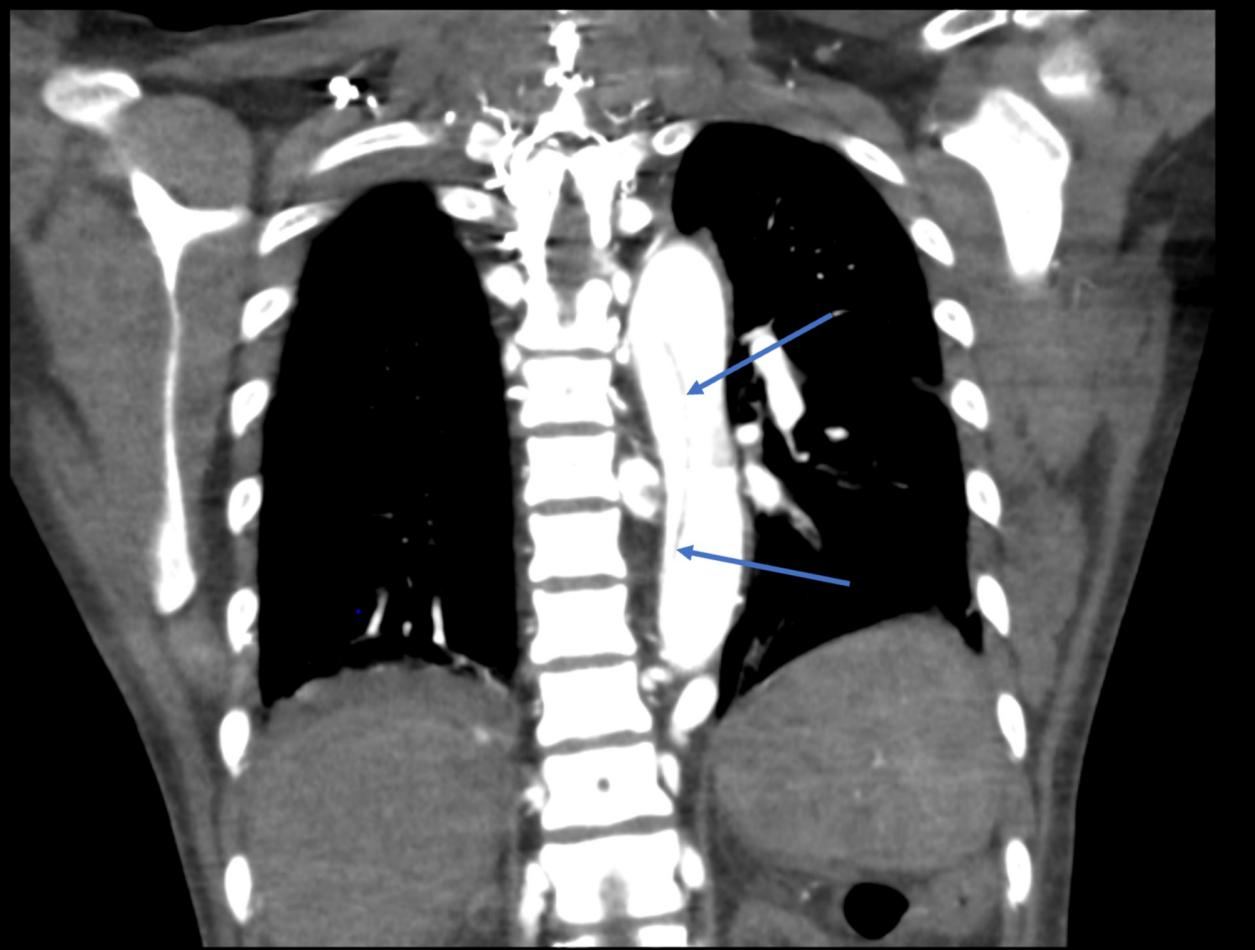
CT chest scan, coronal view, showing aortic dissection
